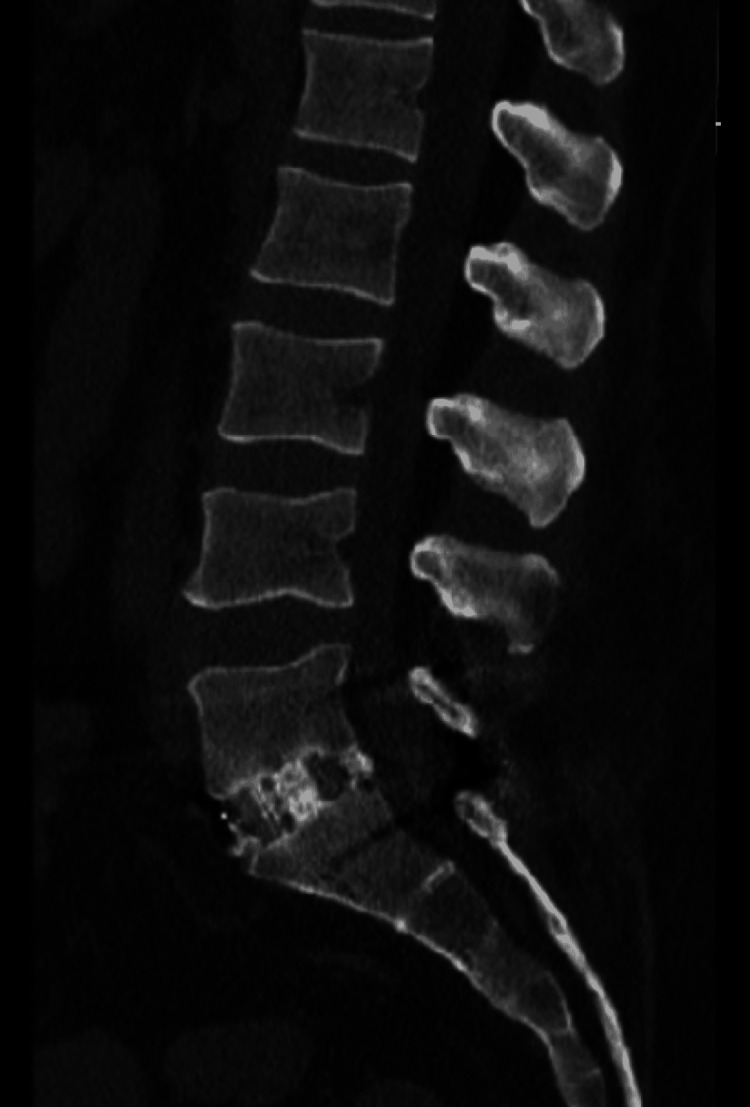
Sagittal CT lumbar spine.
